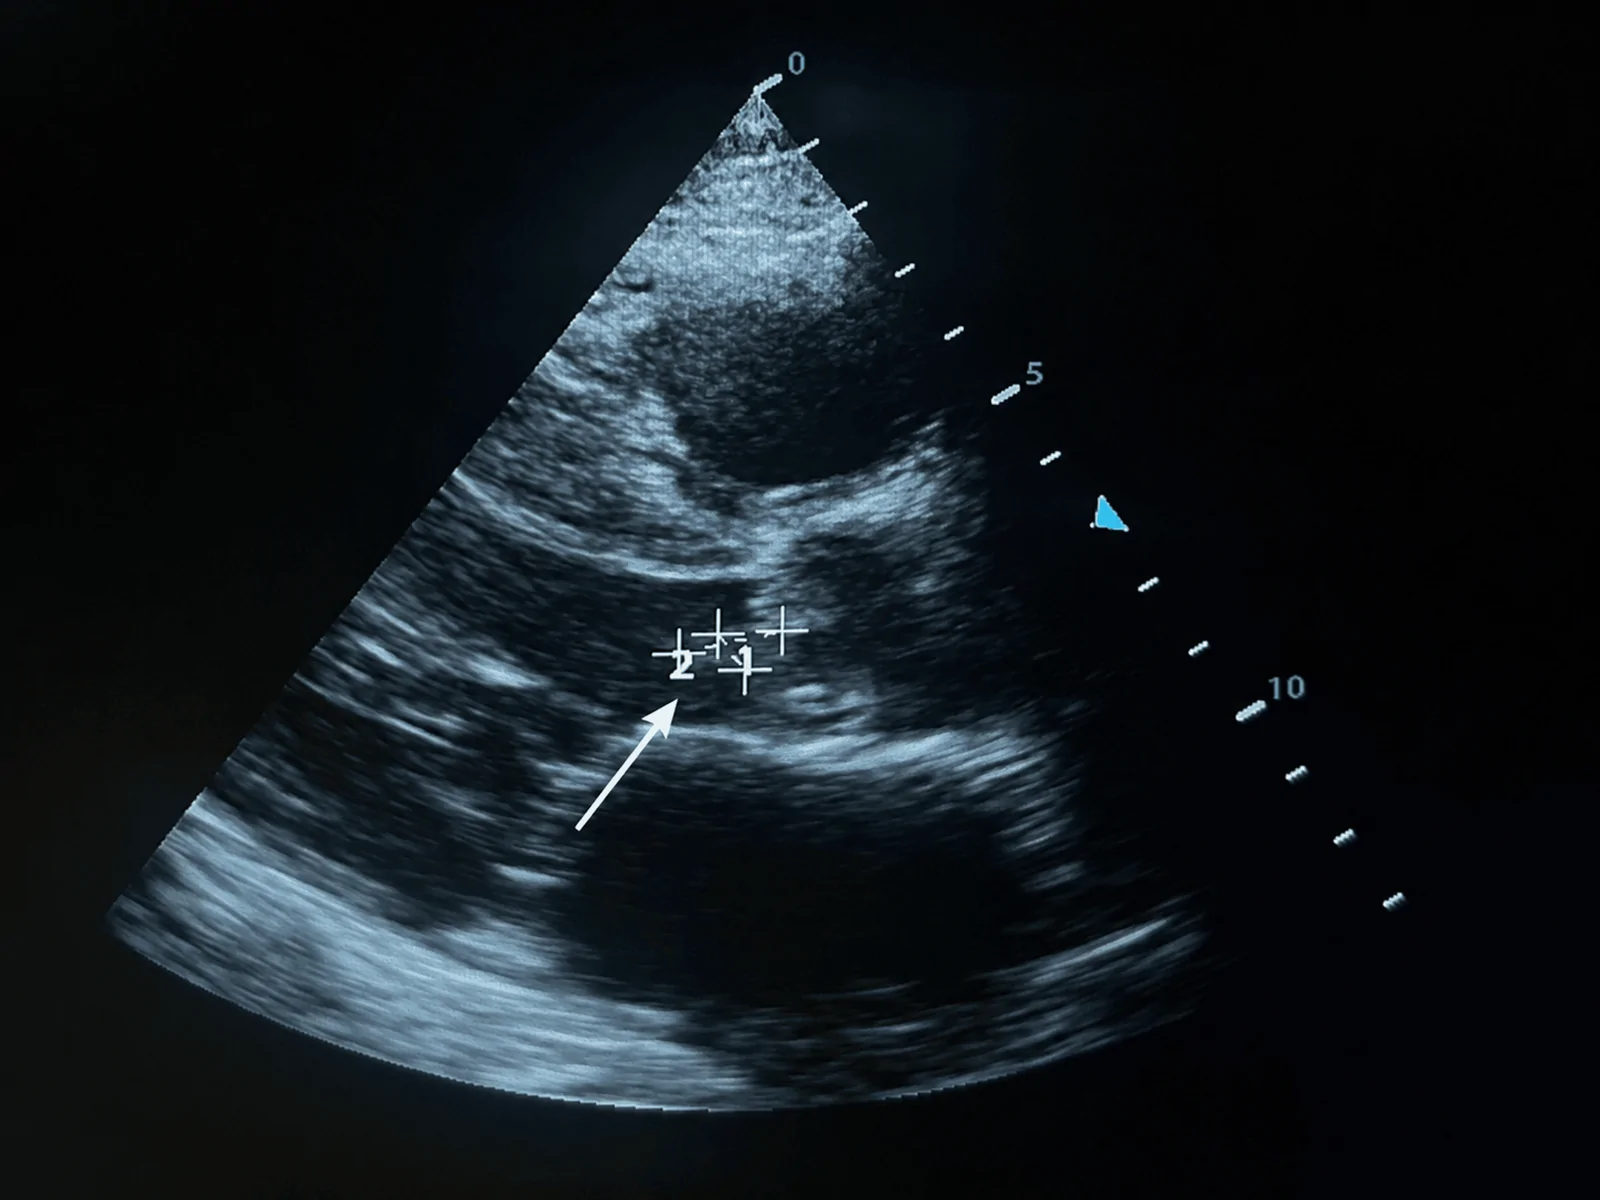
Transthoracic echocardiography showing mitral-valve vegetation in Case 1. A mobile mitral-valve vegetation measuring 12.5 × 5.6 mm is shown. The white arrow indicates the vegetation.
